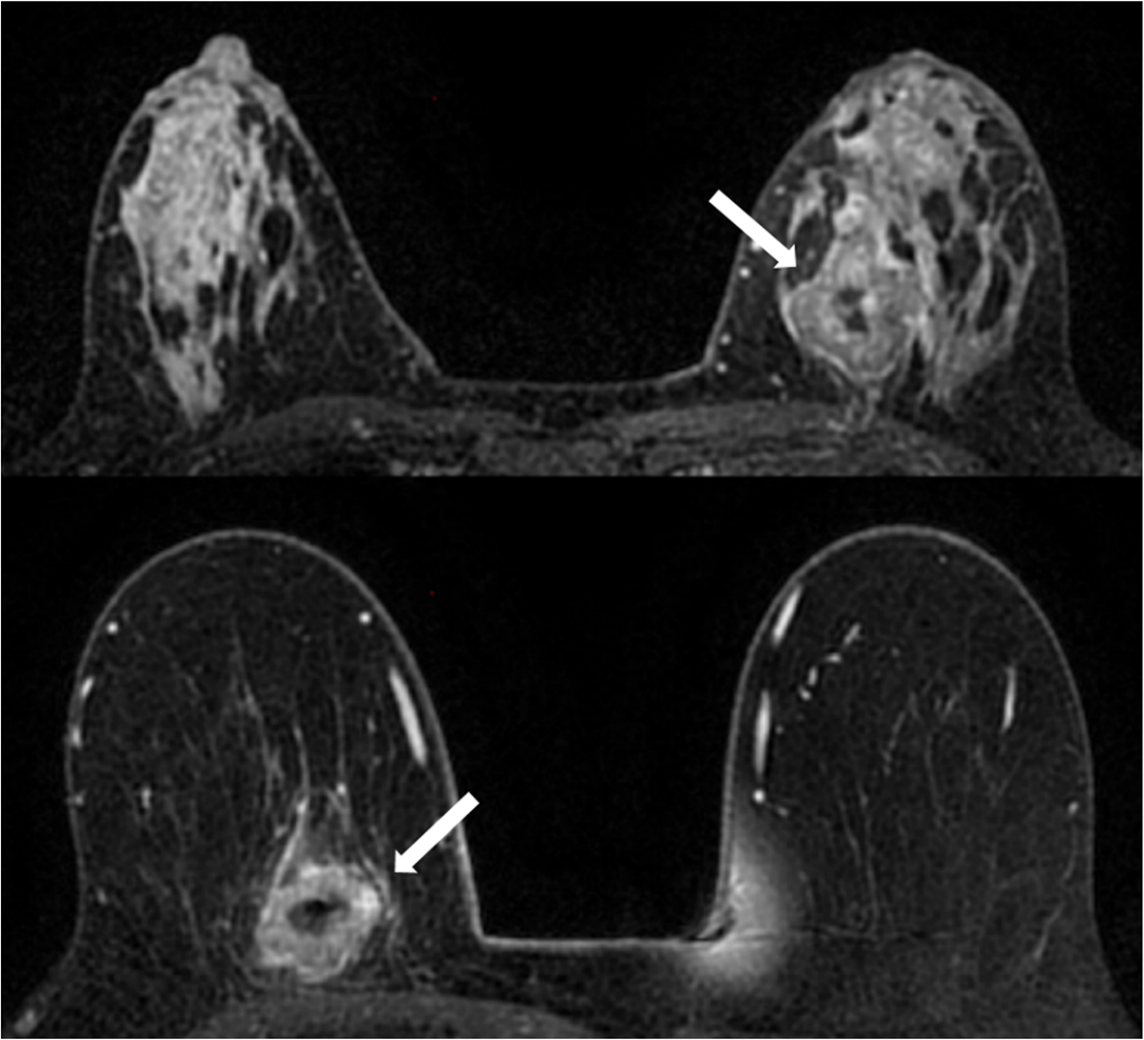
Top: contrast-enhanced fat-saturated T1-weighted image of a 50-year-old patient with a HER2-enriched cancer in the left breast. Bottom: contrast-enhanced T1-weighted image of a 59-year-old patient with a triple-negative cancer in the right breast. Both lesions are irregularly shaped and margined, with heterogeneous contrast enhancement and central necrosis. Radiomic signatures derived from contrast-enhanced MRI (CE-MRI) accurately differentiated HER2-enriched from triple-negative breast cancer with an overall accuracy of 73.5% in our patient collective
